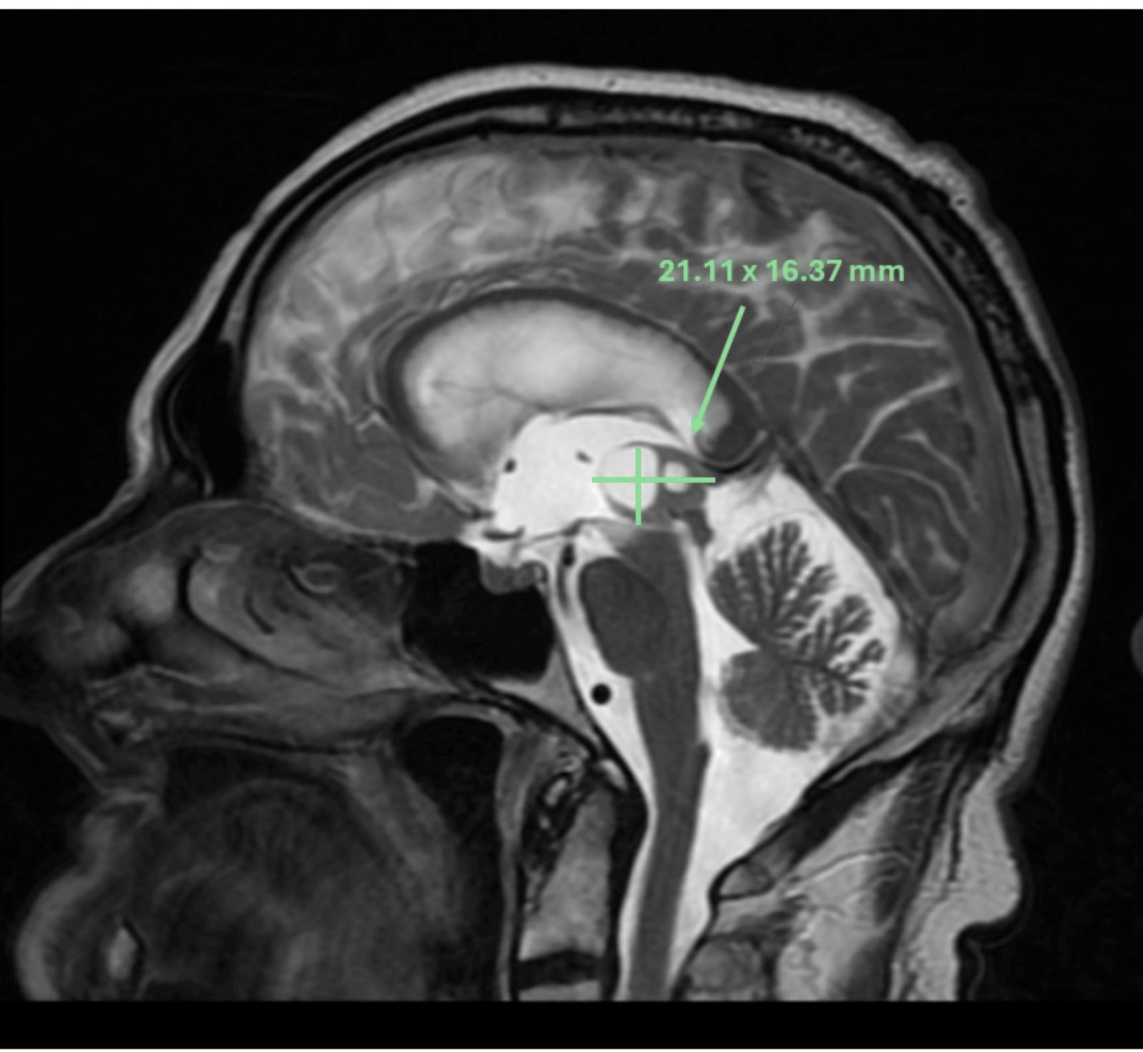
The sagittal T2 MRI head showed a pineal region mass with a hyperintense anterior cystic component.
